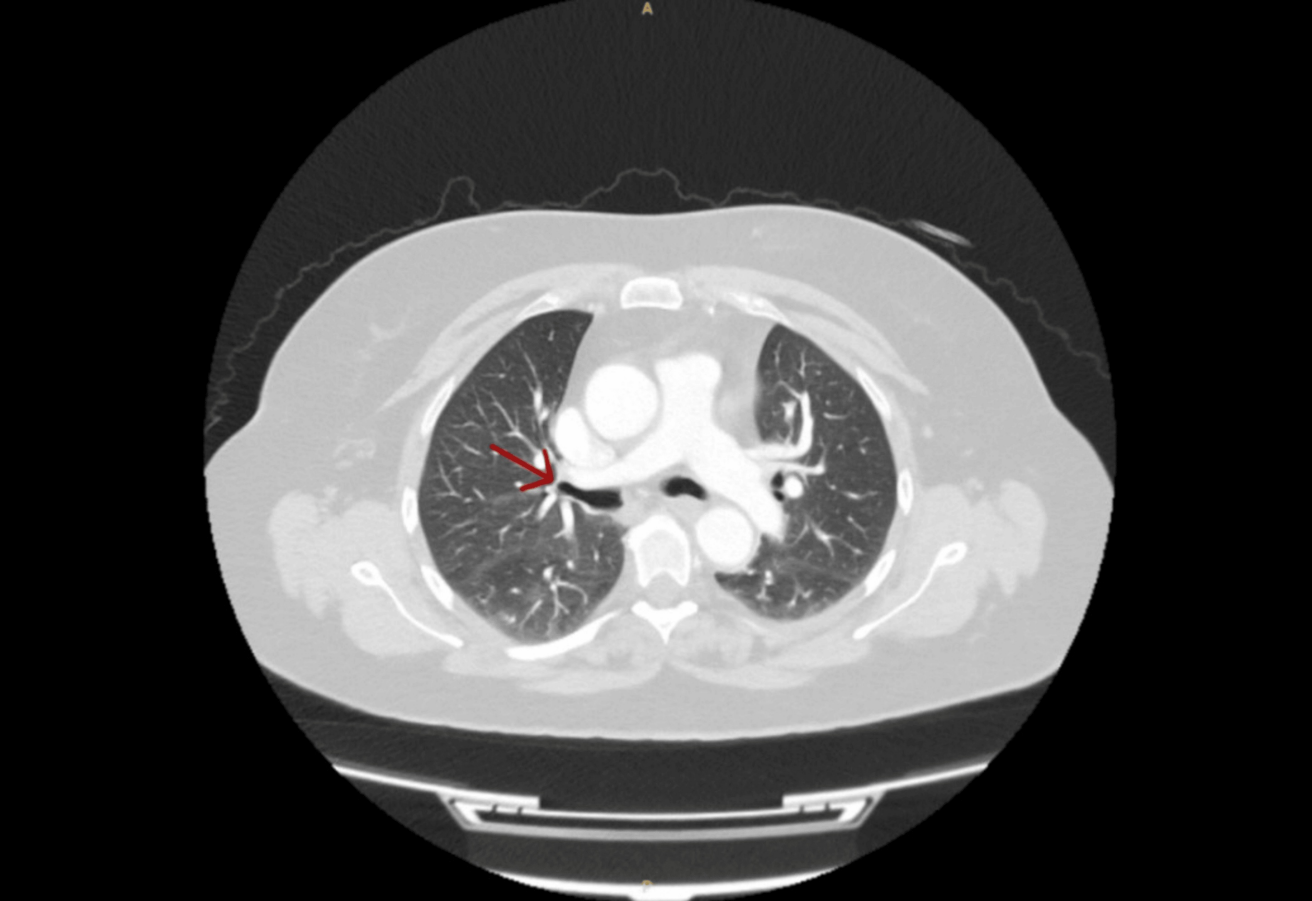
Cross-sectional CT of the chest and abdomen with contrast The red arrow indicates filling defects in the right inferior lobar and several right lower lobe segmental pulmonary artery branches, consistent with PE. Loss of lung volume with multifocal segmental atelectasis is more prominent compared to the previous examination. PE, pulmonary embolism
